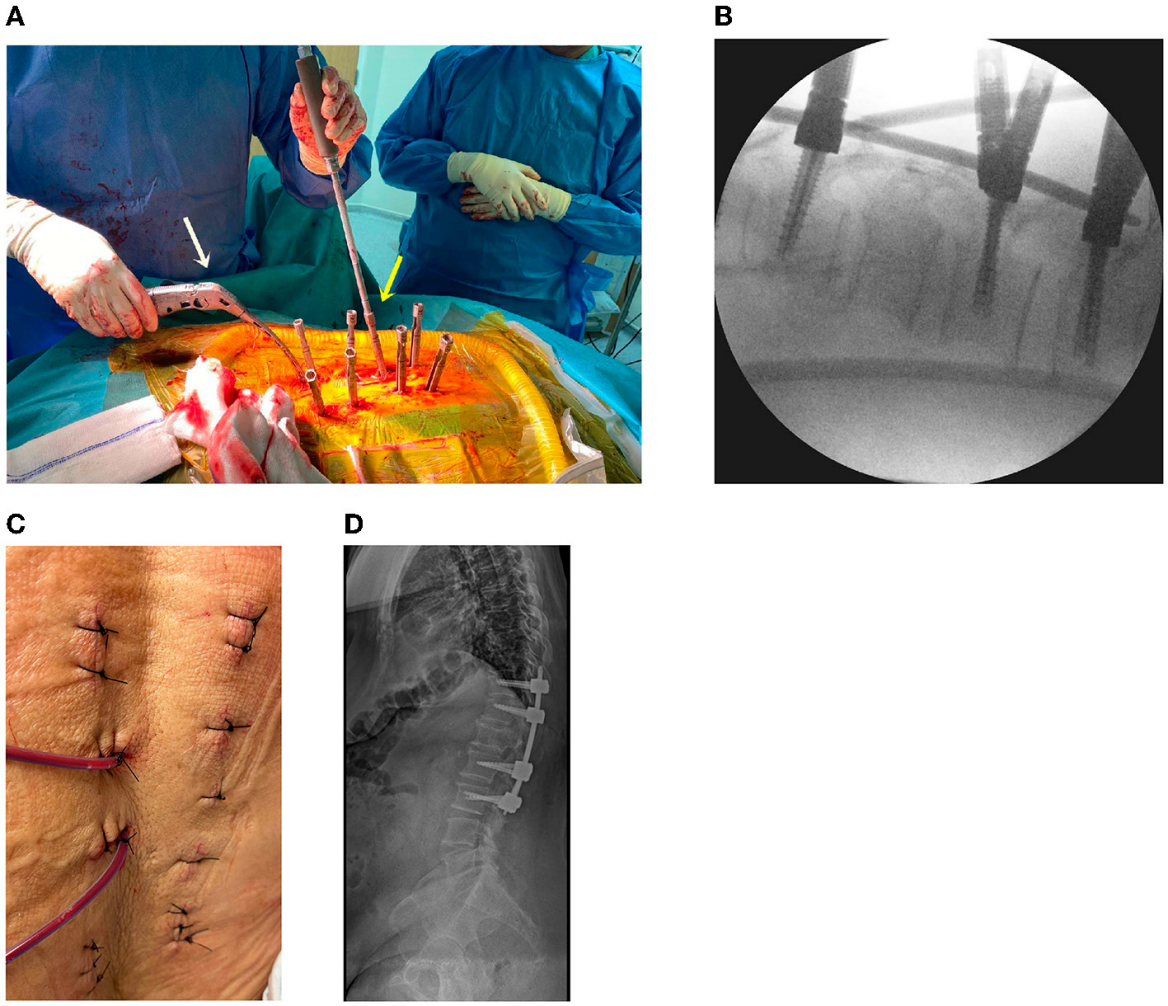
(A) Operative setting using the percutaneous screw fixation procedure. Screw capping (yellow arrow) and rod placement (white arrow) are performed. (B) Intraoperative fluoroscopy for screw and rod application. (C) The skin is sutured after the surgery. (D) Plain radiograph after the surgery.
